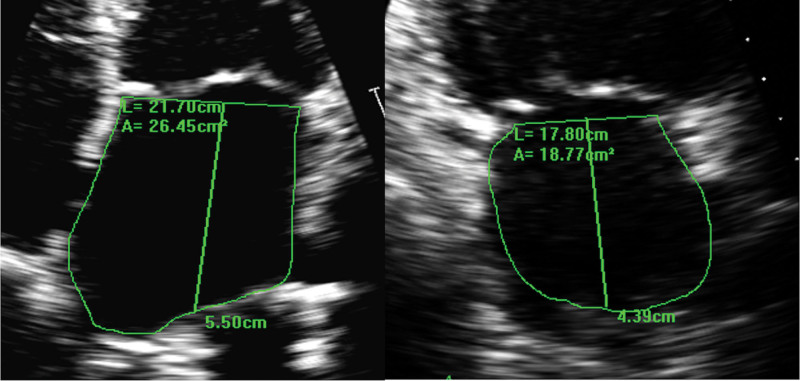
Left atrial (LA) volume measurement using the biplane area-length method. Zoomed view of the LA in the apical 4-chamber view is on the left and apical 2-chamber view on the right. A indicates area; and L, length.
